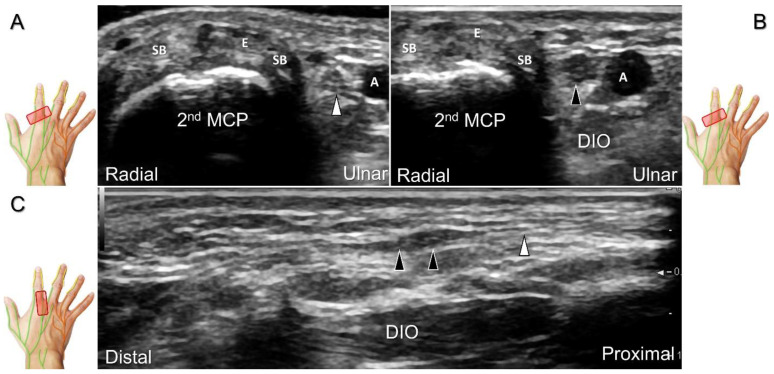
Sonographic imaging (short-axis view) for a neuroma of the dorsal common digital nerve, proximal site (A) and the site of the lesion (B). Long-axis imaging of the nerve/neuroma (C). White arrowhead: normal dorsal common digital nerve; black arrowheads: neuroma. SB: sagittal band; E: extensor tendon; MCP: metacarpal bone; DIO: dorsal interosseous muscle; A: artery.
